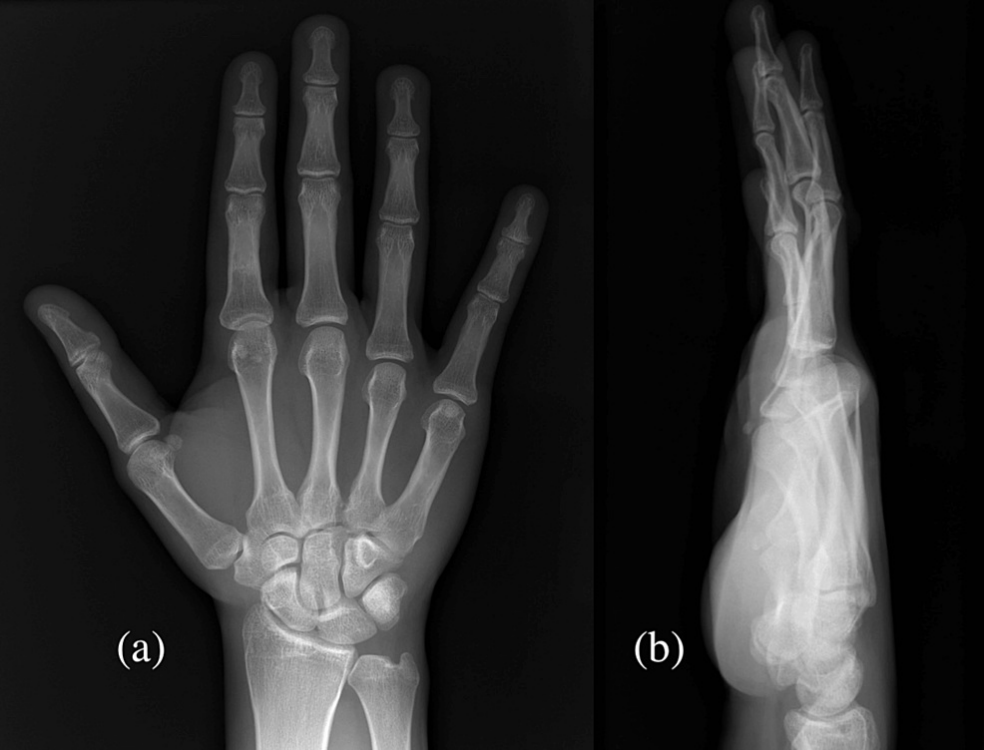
Follow-up X-ray five weeks post injury. (a) Anteroposterior view and (b) lateral view.
